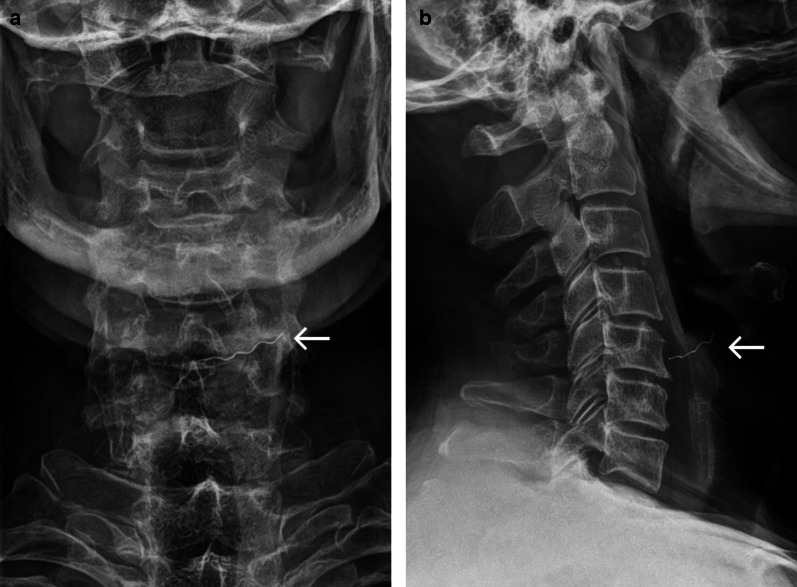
Metal wire. Anteroposterior (a) and lateral (b) radiographs showing a thin radiopaque structure (arrow) in projection over the hypopharynx anterior to the 5th cervical vertebrae
